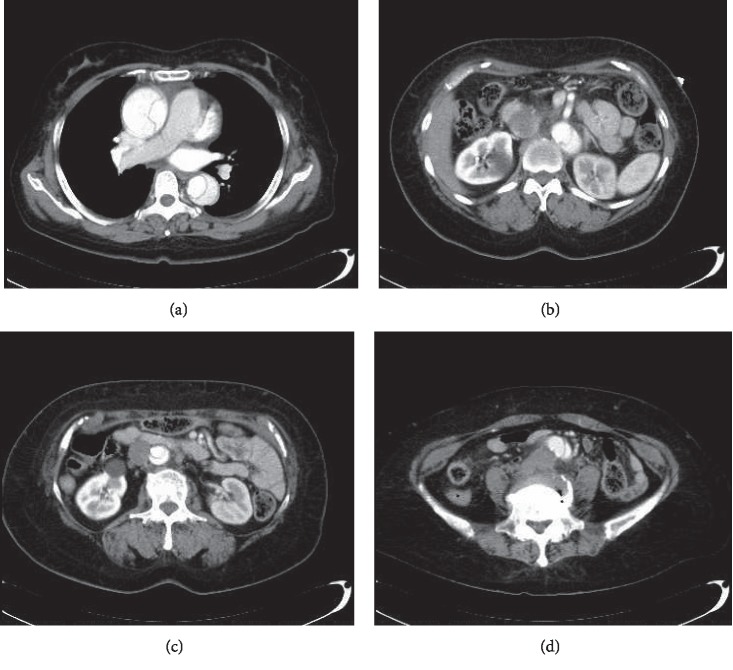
Preoperative enhanced computed tomography (CT) image shows (a) acute type A aortic dissection, (b) superior mesenteric artery perfusion from the true lumen, (c) left kidney not well enhanced, and (d) dissected lumen extending to the terminal aorta.
